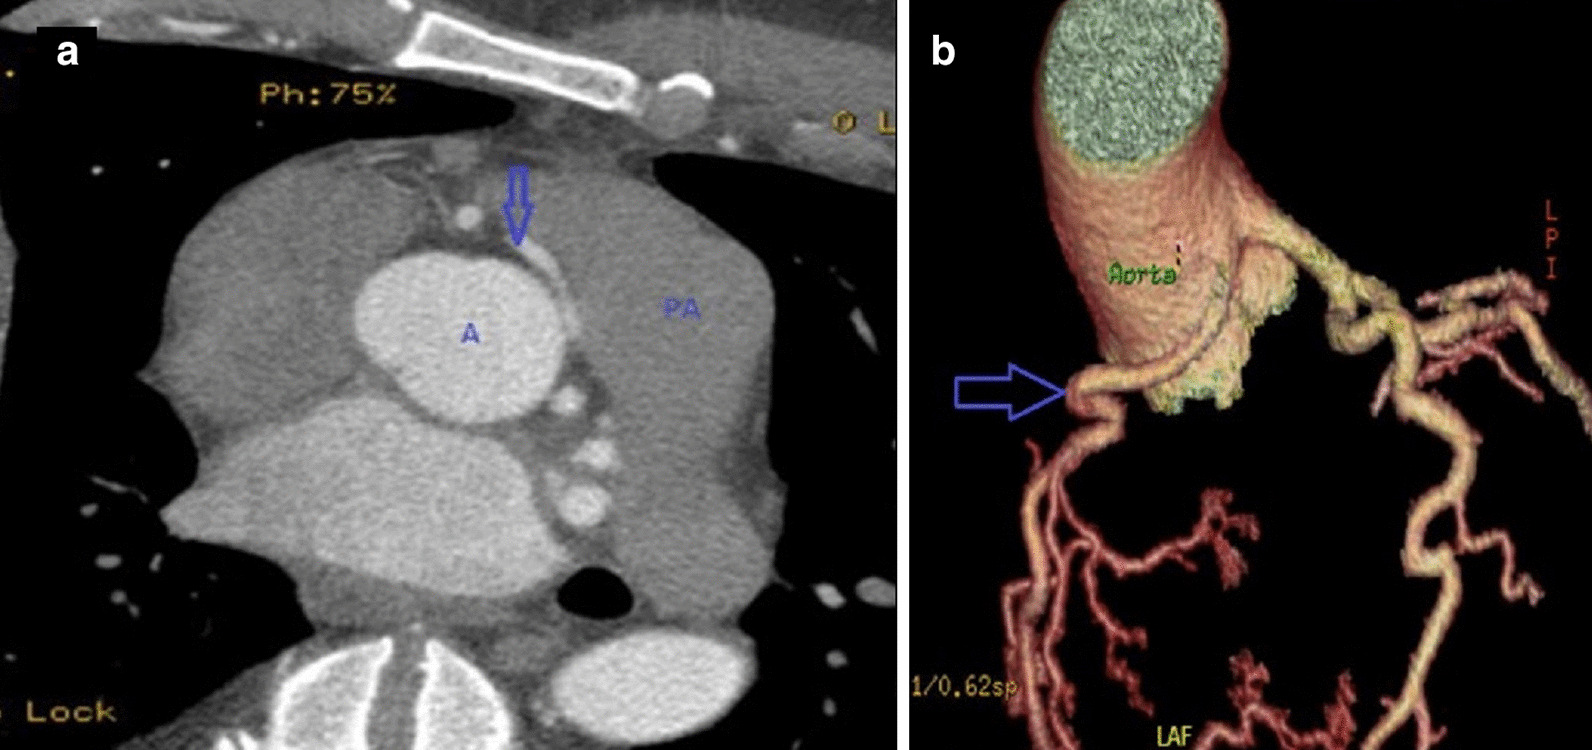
Case 6. Coronary CT angiogram (CTA). a Axial CTA with anomalous right coronary artery (blue arrow) with left coronary cusp origin just anterior to the origin of the left main coronary artery. b Volume rendered CTA with anomalous right coronary artery (blue arrow)
